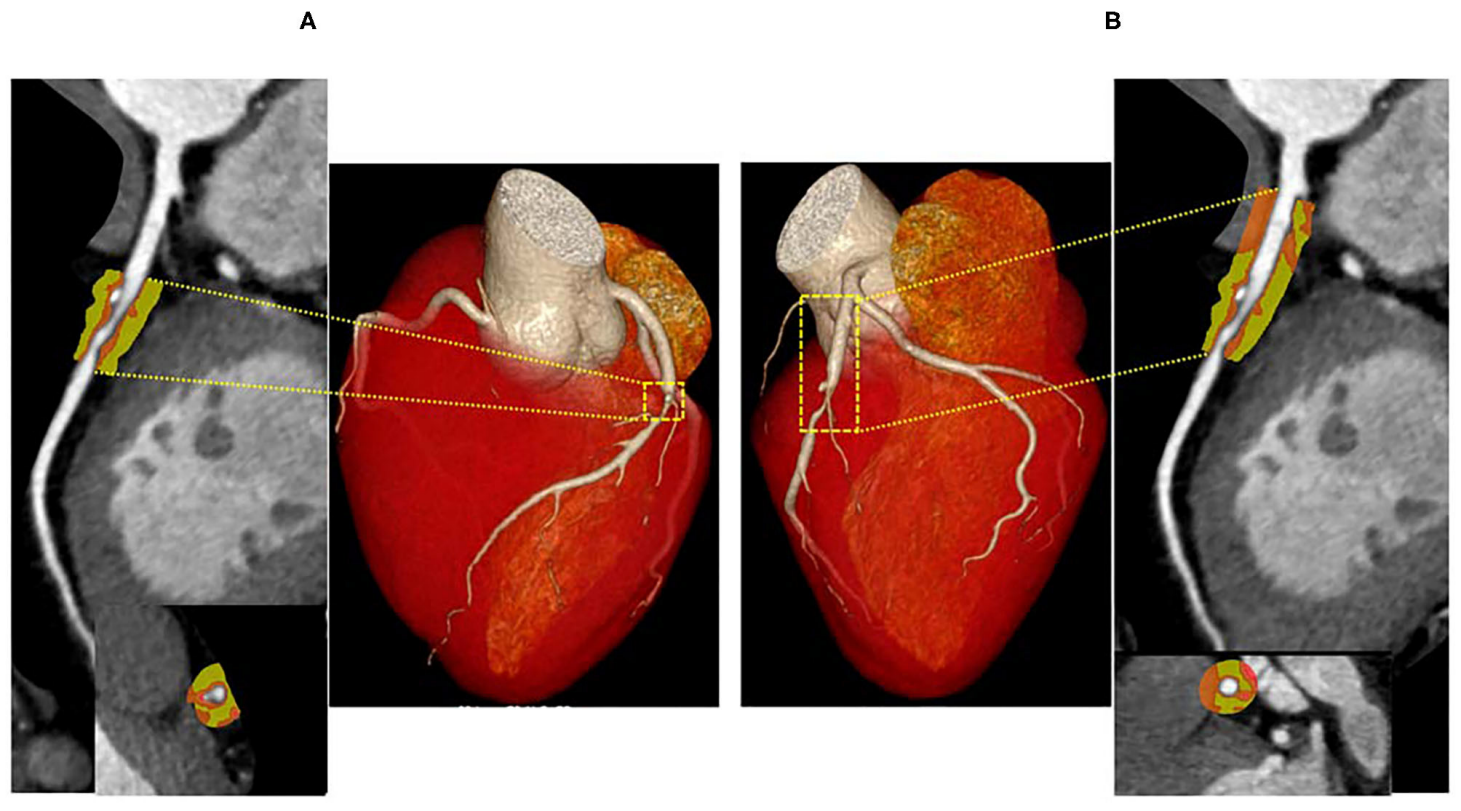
Representative CCTA image of PCAT around LAD. A hemodynamically significant stenosis (FFR = 0.73) in the middle segment of LAD. PCAT CT attenuation was defined as the mean CT attenuated value within a radial distance equal to the diameter of the vessel. The length of the lesion-based PCAT was defined from the proximal to the distal shoulder of the lesion (A), the vessel-based PCAT was focused on the proximal 40-mm segments of all three major epicardial coronary vessels (10 to 50 mm from right coronary artery ostium) (B). LAD, left anterior descending artery; PCAT, pericoronary adipose tissue.
